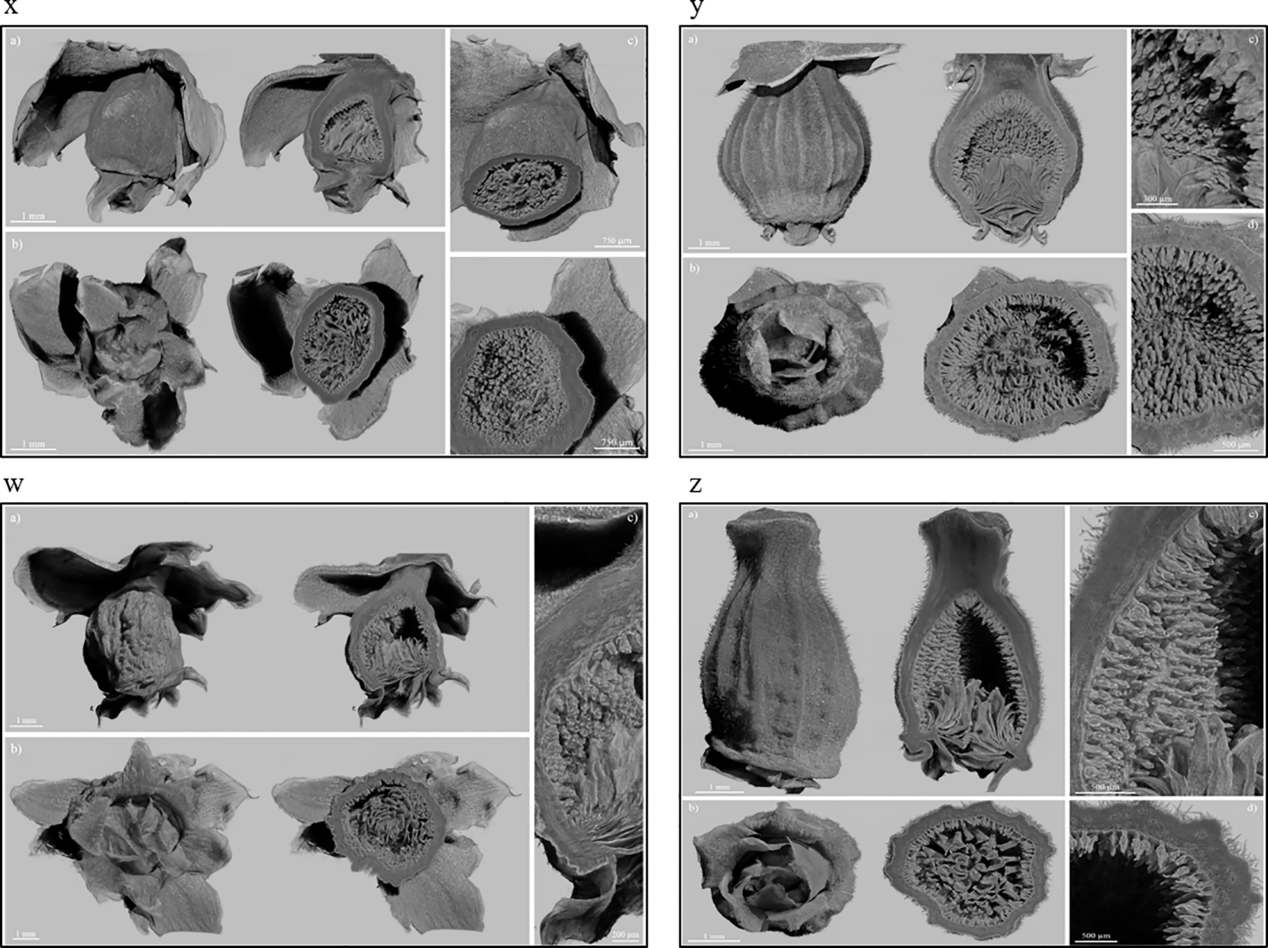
Fruits of Dottato, breba (x) and main crop (y), and Petrelli, breba (w) and main crop (z), as obtained by X-ray analysis. For each panel, subfigures a) and b) show the frontal and bottom views, respectively, with related internal cross sections; subfigures c) and d) show internal details.
